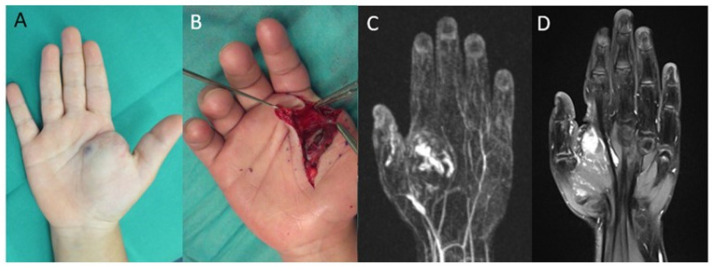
Right hand of a five-year-old female patient with an arterio-venous malformation of the right hand with diffuse intermuscular invasion: (A) blue discoloration and swelling in the palm and thenar region of the right hand; (B) intraoperative image of the arterio-venous malformation; (C) contrast-enhanced MR-angiography shows an arterio-venous malformation of the palm and thenar region of the right hand; (D) MRI of a recurrence of the arterio-venous malformation 6 years after surgical resection.
